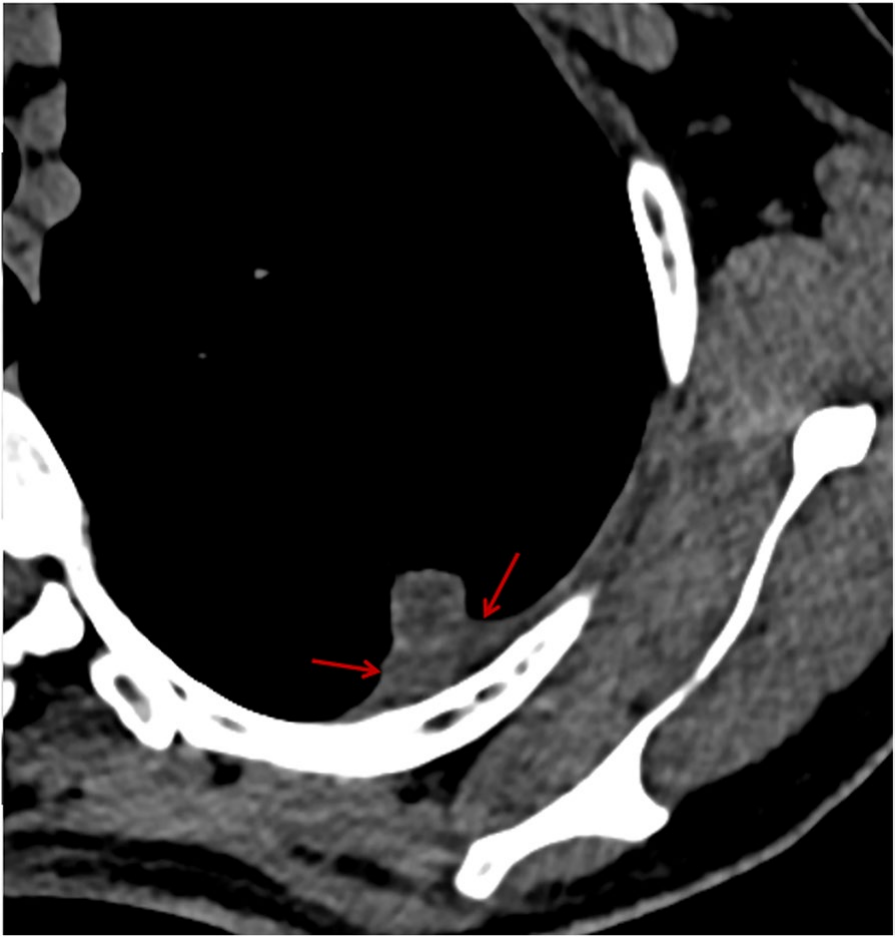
SPAN with type III of nodule-pleura relationship. A 48-year-old woman with left upper lobe SPAN which is confirmed as a benign lesion during follow-up. On the axial CT image, a part of the nodule with slightly high density embeds in the significantly thickened pleura (red arrows)
